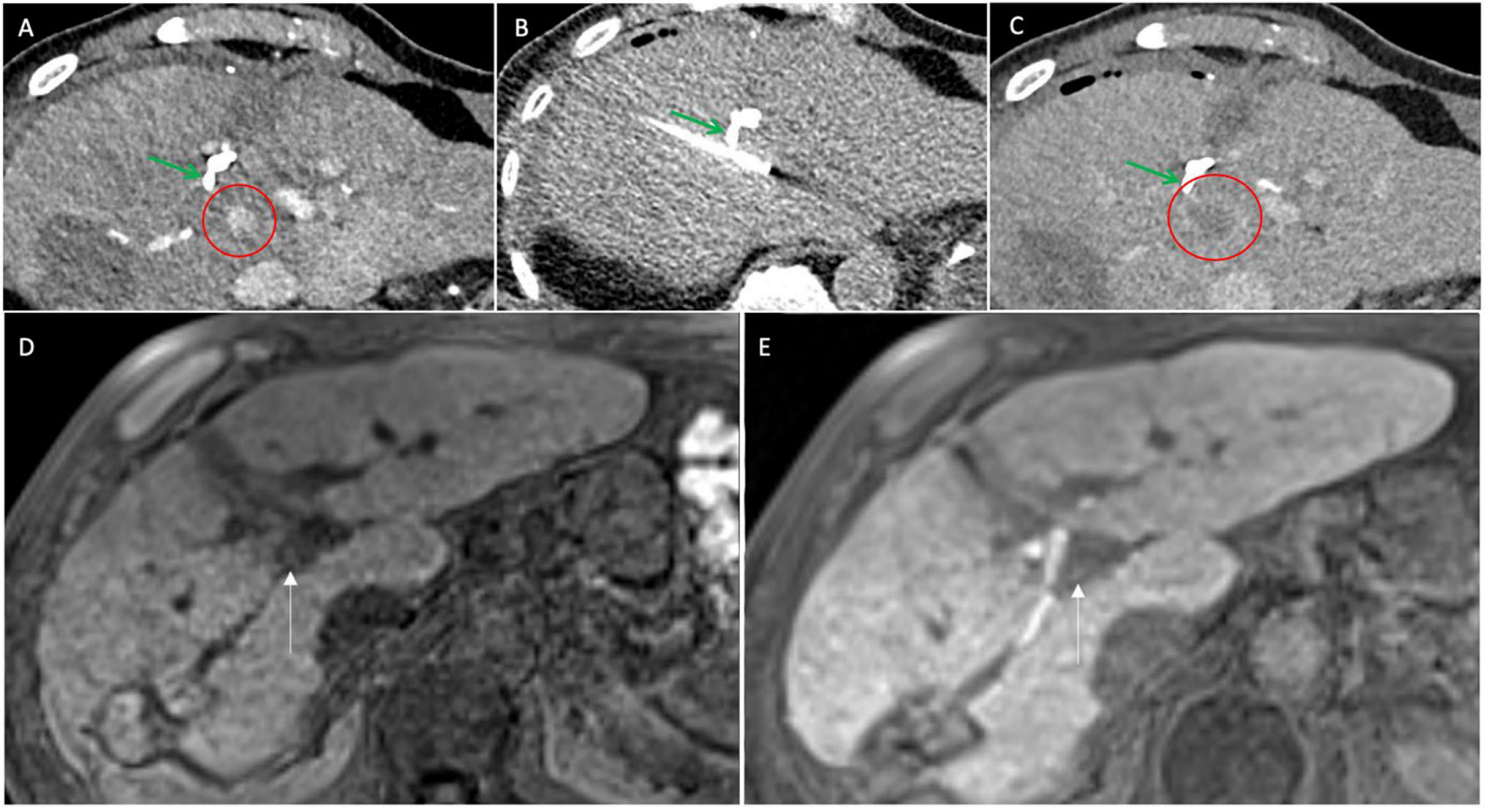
CT-guided cryoablation of patient 2 with HCC near the right hepatic duct and 30 months follow-up MRI. A: Pre-ablation planning CT (axial in portal venous phase) shows a 11-mm HCC with early contrast enhancement next to the right hepatic duct with a minimum distance of 5 mm. B: Position of the cryoprobe just before ablation (CT axial without contrast medium). C: Post-ablation CT (axial in portal venous phase) shows complete necrosis of the HCC with inflammatory rim enhancement. D, E: Small focus of hemorrhage with settle hyperintensity on pre- and post-contrast T1-weighted MRI (axial), but no enhancement 30 months post-ablation, indicating no residual or recurrent HCC. Previous follow-up (not shown) revealed a larger area of hemorrhage that decreased over time. *red circle: tumor/post-ablation necrosis; green arrow: bile duct; white arrow: follow-up area at the site of the previous HCC. *HCC: Hepatocellular carcinoma
